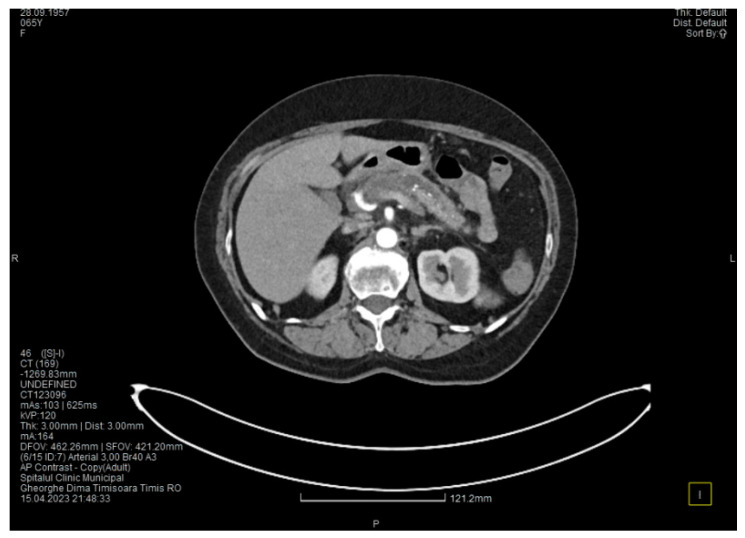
The initial CT scan shows a dilated Wirsung duct and calcifications in the pancreatic tissue.
